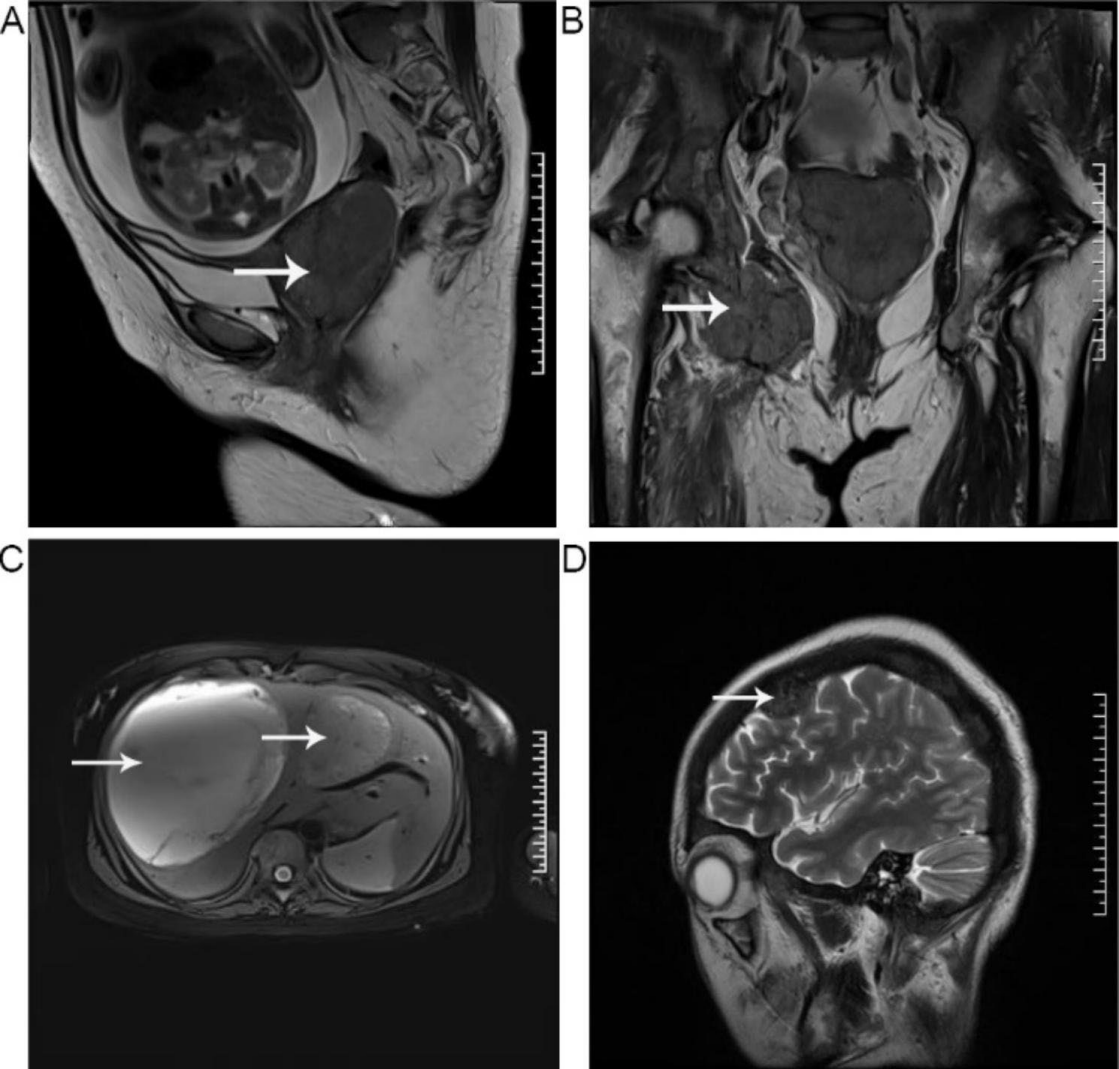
Magnetic Resonance Imaging of tumor and metastases. (A) Cervix. (B) Ilium and ischium. (C) Liver. (D) Brain
